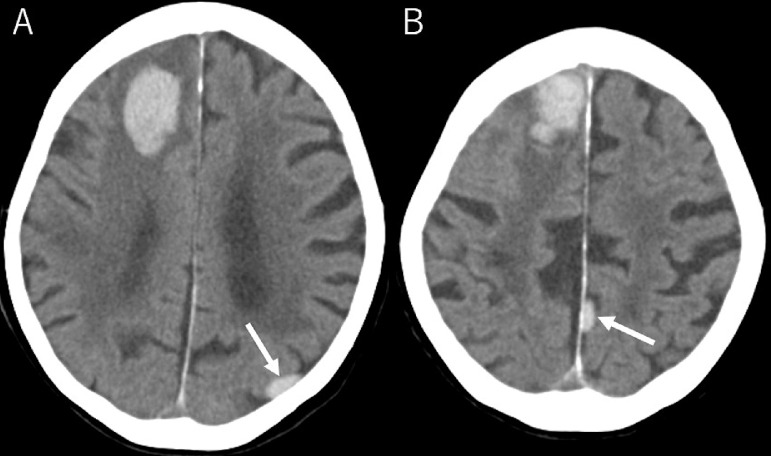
(A, B) Non-contrast coronal computed tomography scans showing small subdural hematomas and in the acute phase in the left parietal convexity and interhemispheric region (*arrow*).
